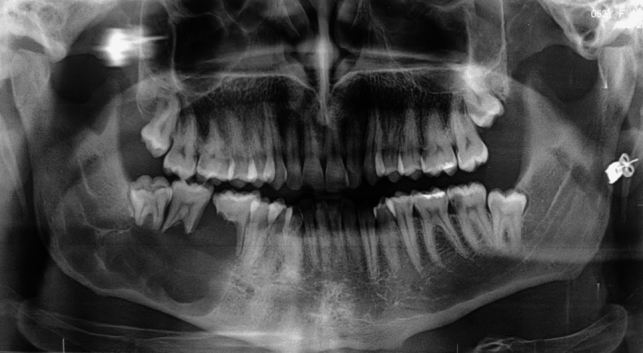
Panoramic radiography shows the lytic lesion in the right jaw
